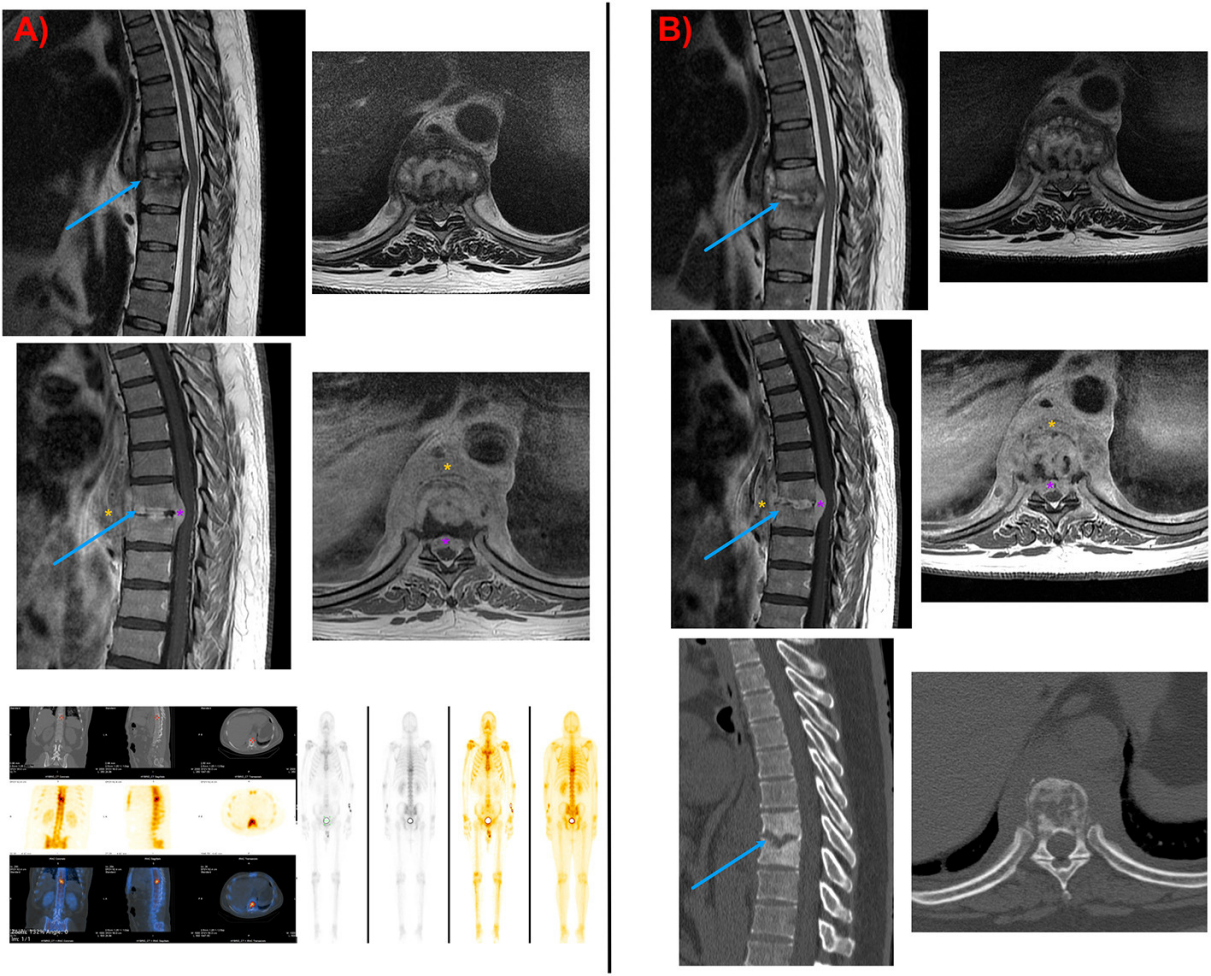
(A) Initial MRI image. Note the important prevertebral mass with disc and adjacent bone involvement typical of fungal spondylodiscitis. In addition, an epidural mass was seen with spinal cord compression. Positive SPECT-TC and gamma scan showing a pathological uptake where spondylodiscitis was suspected. (B) MRI and CT were taken a few days before surgery. The results reaffirmed the necessity of surgery in this case, given the inefficiency of medical treatment. Note the worsening of the Kyphosis curve, the damaged bone, and the epidural mass. Blue arrow, spondylodiscitis destruction; Orange asterisk, prevertebral mass infection; Purple asterisk, epidural mass infection.
